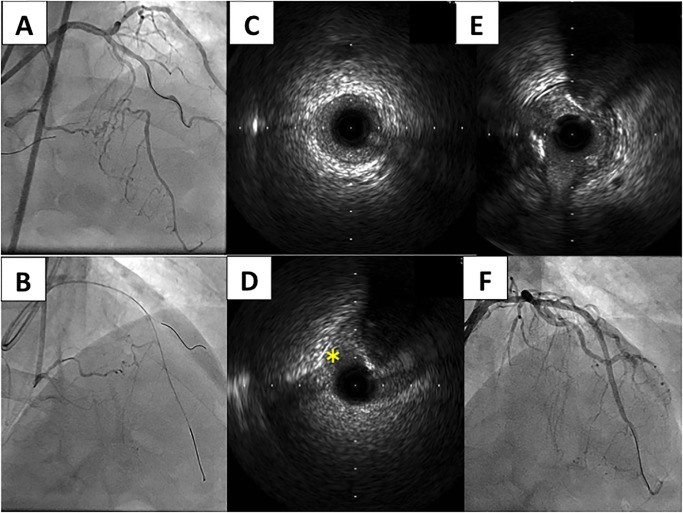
Angiogram and intravascular ultrasound findings of one case in the antegrade true sub-true group. Panel A: Bidirectional coronary angiogram before PCI. Panel B: A stiff guidewire (Conquest Pro 8–20) successfully crossed by antegrade IVUS guide wiring. Panel C: An IVUS image at CTO distal revealed that the guidewire was within the true lumen. Panel D: An IVUS image at CTO revealed that the guidewire was in subintimal space (*). Panel E: An IVUS image at CTO proximal revealed that the guidewire was within the true lumen. Panel F: Final angiogram.
